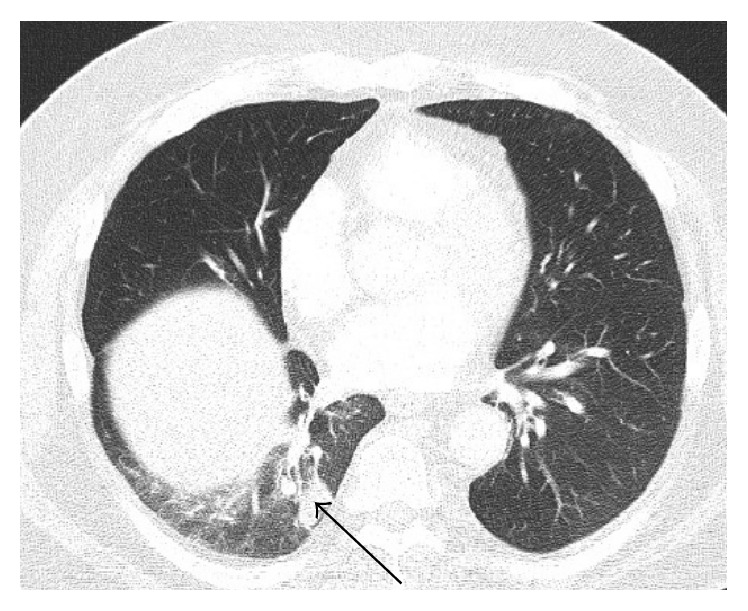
Axial image of CT of chest performed on January 31, 2014: pulmonary windows demonstrating a 2.3 cm nodular opacity in the right lower lobe (arrow) with minimal subsegmental atelectatic changes and ground-glass opacity.
